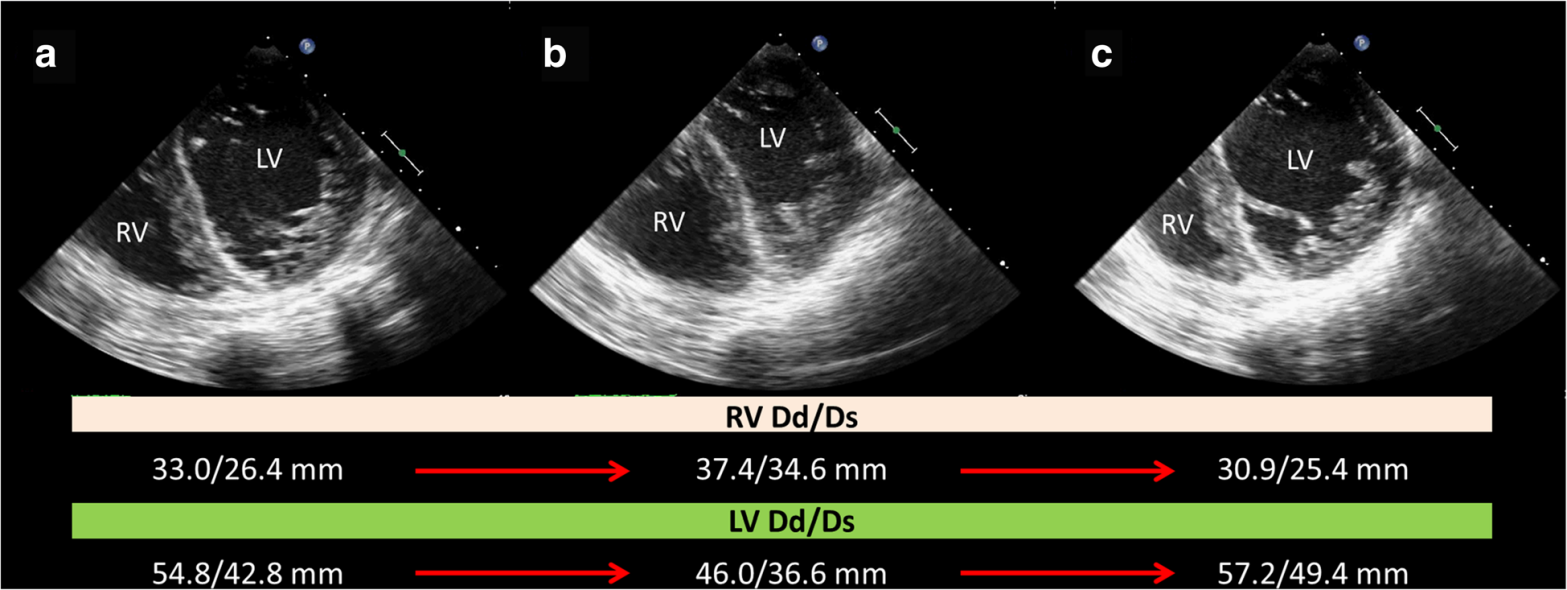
Mid-esophageal four-chamber TEE images collected during the procedure. a TEE image after THV deployment showing normal RV size (RV Dd/Ds = 33.0/26.4 mm). b TEE image collected 2 min after protamine administration demonstrating RV dilatation (*yellow arrow*) (RV Dd/Ds = 37.4/34.6 mm) and left ventricular (LV Dd/Ds = 46.0/36.6 mm) volume reduction. Severe systemic hypotension was observed at the same time. c TEE image after 1 min of chest compressions (approximately 5 min after protamine administration) demonstrating that the RV and LV size and wall motion had returned to baseline (RV Dd/Ds = 30.9/25.4 mm, LV Dd/Ds = 57.2/49.4 mm). *Dd* dimension end-diastole, *Ds* dimension end-systole, *LV* left ventricle, *RV* right ventricle, *TEE* transesophageal echocardiography
